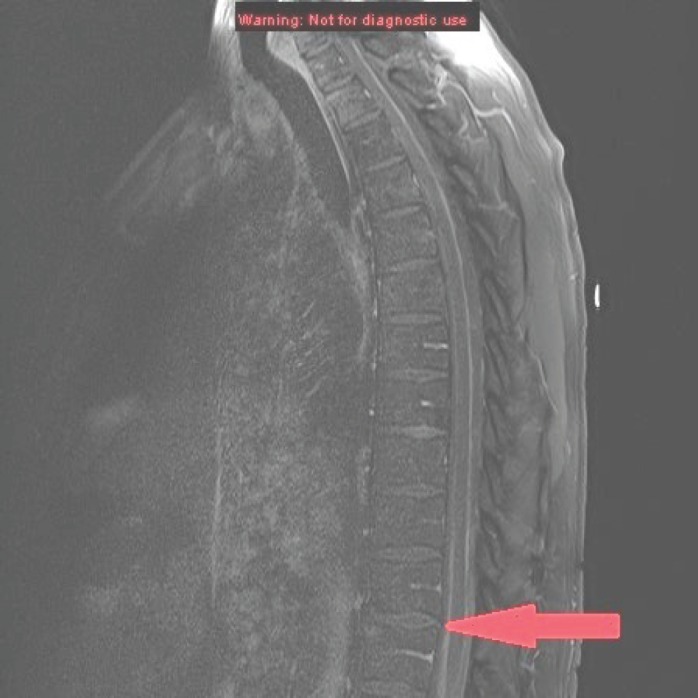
MRI of the spine sagittal section showing anterior and posterior parallel thick lines of avid enhancement corresponding to the leptomeninges, which is highly abnormal and indicates leptomeningeal carcinomatosis.
